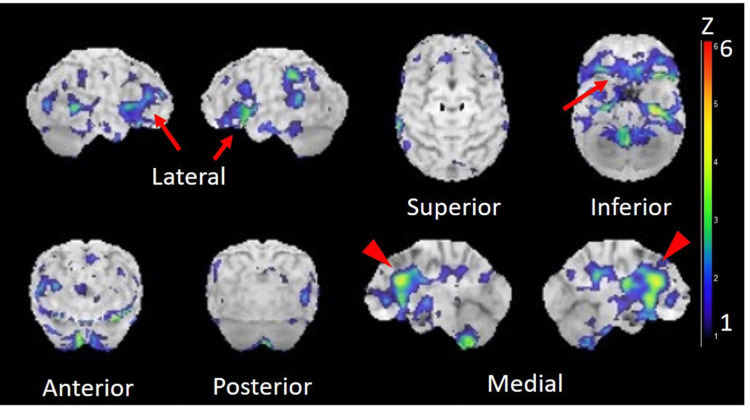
SPECT showing hypoperfusion in cortices Cerebral blood flow single-photon emission computed tomography using N-isopropyl-p-[123I] iodoamphetamine (IMP-SPECT) reveals hypoperfusion in cortices including the bilateral inferior frontal, orbital (arrows) and anterior cingulate gyri (arrowhead). The Z-score increases as the degree of the decrease in cerebral blood flow are larger than that of an age-matched normal database.
